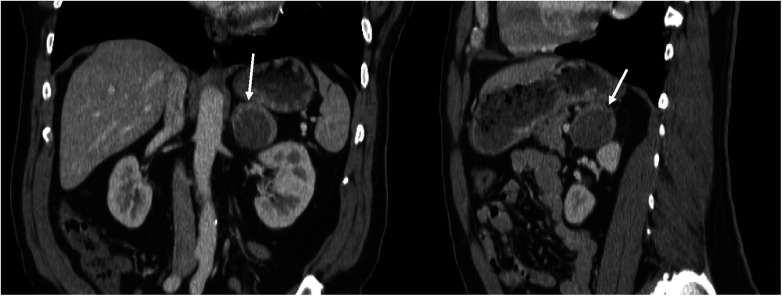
Coronal and sagittal views of CT abdomen with contrast, showing 4.4 cm left adrenal mass with peripheral enhancement and central heterogeneous hypoenhancement, July 2022.
